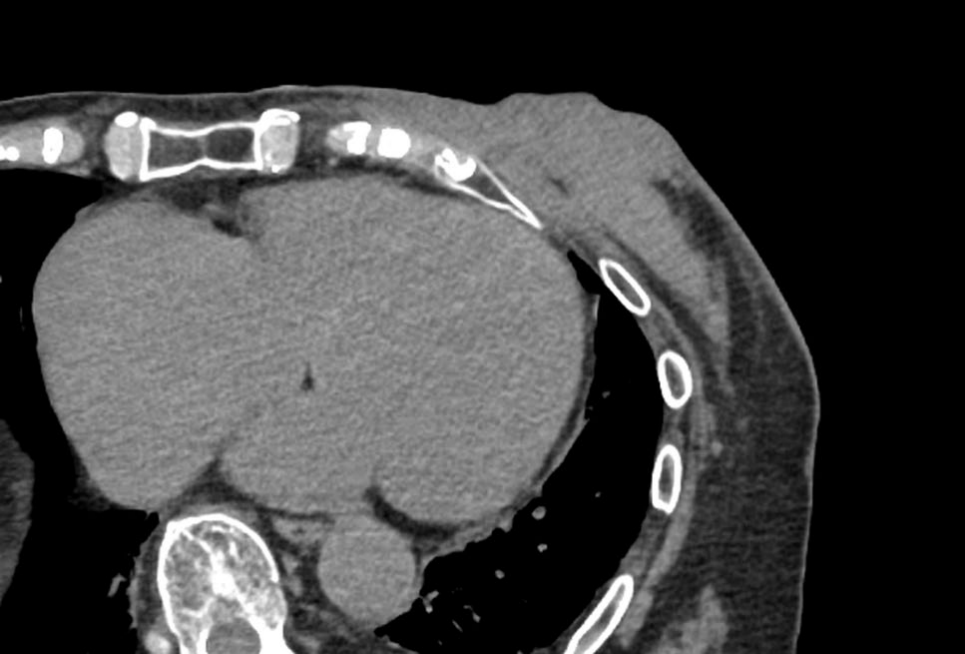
CT scan at eight months after the completion of re-irradiation
